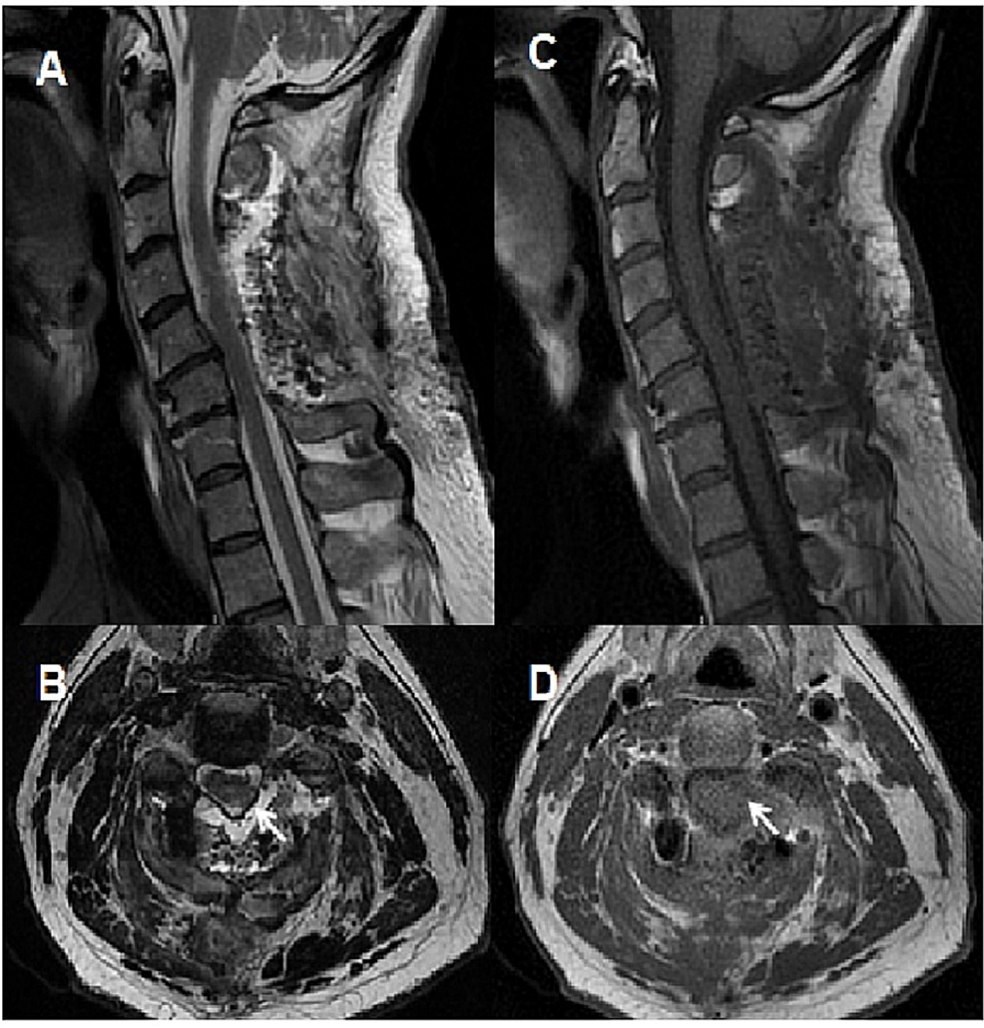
Post operative MRI of the cervical spine Post-operative MRI of the cervical spine demonstrating completed resection of the tumor with a cavity (white arrow). (A) Sagittal view of a T2-weighted image; (B) axial view of a T2-weighted image; (C) sagittal view of a T1-weighted image; (D) axial view of a T1-weighted image.
